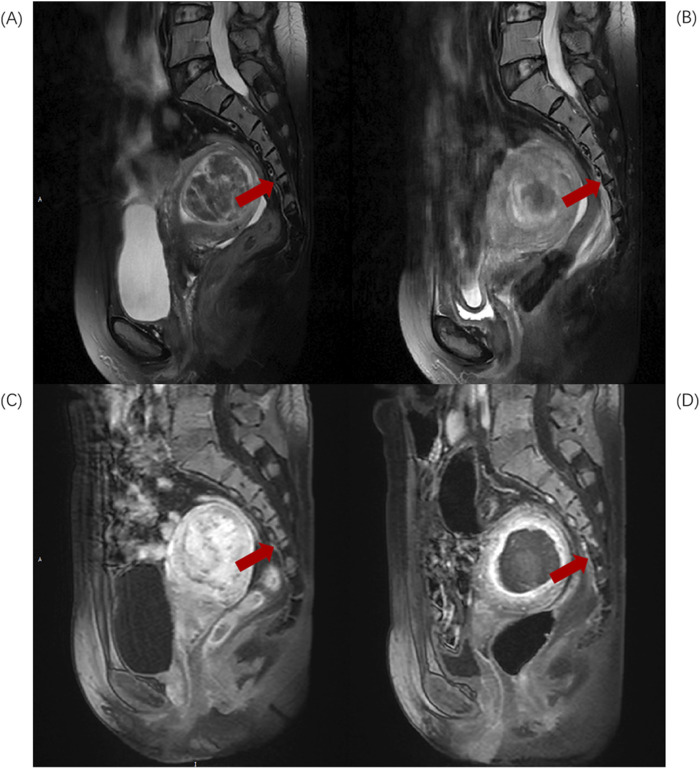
Sagittal MR images of a 46-year-old patient with uterine fibroids before and after HIFU ablation. (A) Pre-HIFU T2WI image shows normal signal intensity in S4 and S5 (arrows); (B) Post-HIFU T2WI shows patchy mixed low signal areas in S4 and S5 (arrows); (C) Pre-HIFU enhanced imaging shows normal enhancement in S4 and S5 (arrows); (D) Post-HIFU enhanced image shows decreased perfusion in the mixed low signal area of S4 and S5 on T2WI (arrow).
